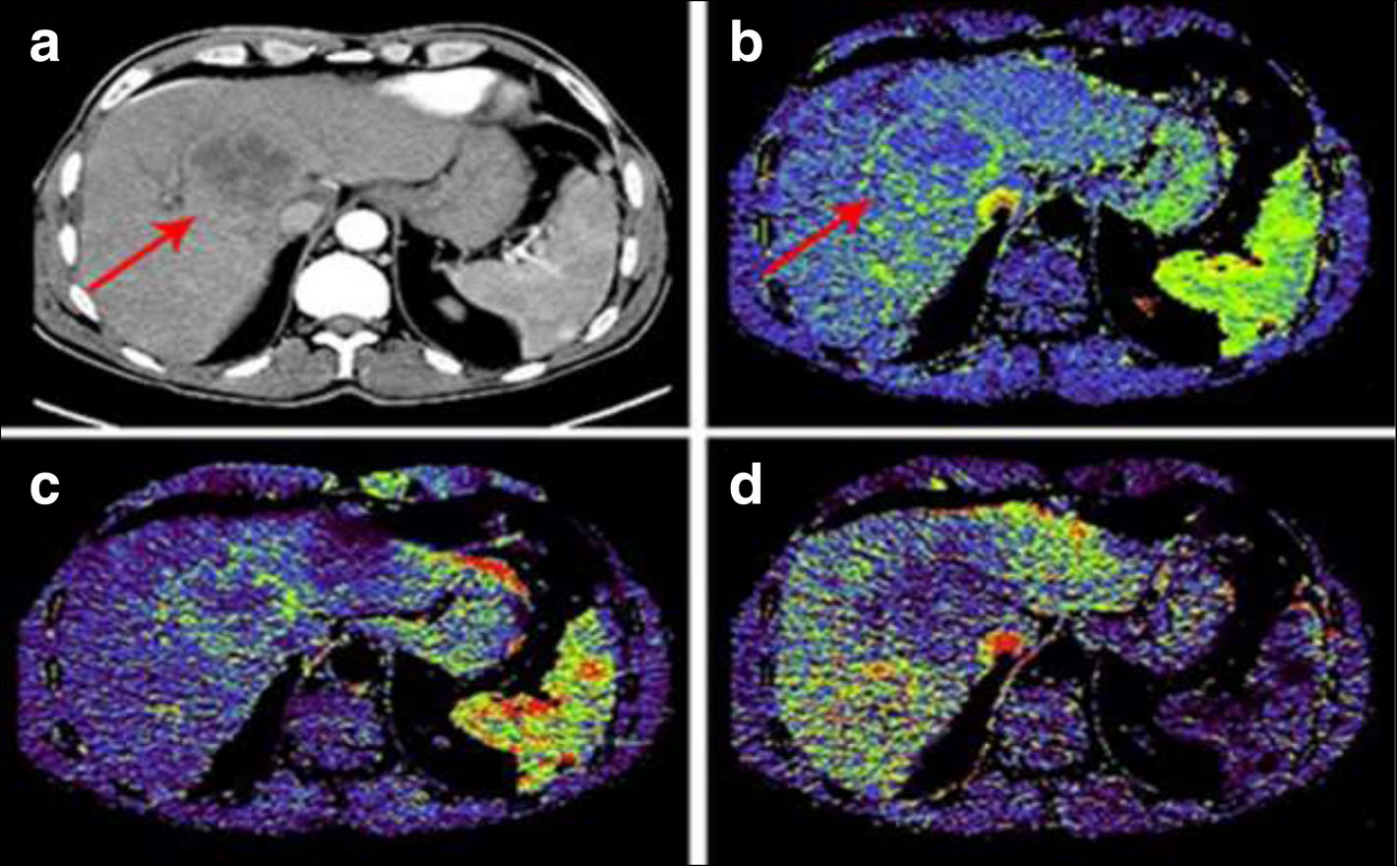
CT-perfusion in moderately differentiated hepatocellular carcinoma. Patient P. Moderately differentiated hepatocellular carcinoma. Images of axial sections of CT with intravenous contrast (arterial phase) (a), perfusion maps BV (b), ALP (c), PVP (d). Nodal formation in the right lobe of the liver (red arrows) unevenly accumulates CA (a). According to the CTP data, an increase in the volume of arterial blood flow along the periphery of the tumor (b, c) is detected, a decrease in portal blood flow (d)
